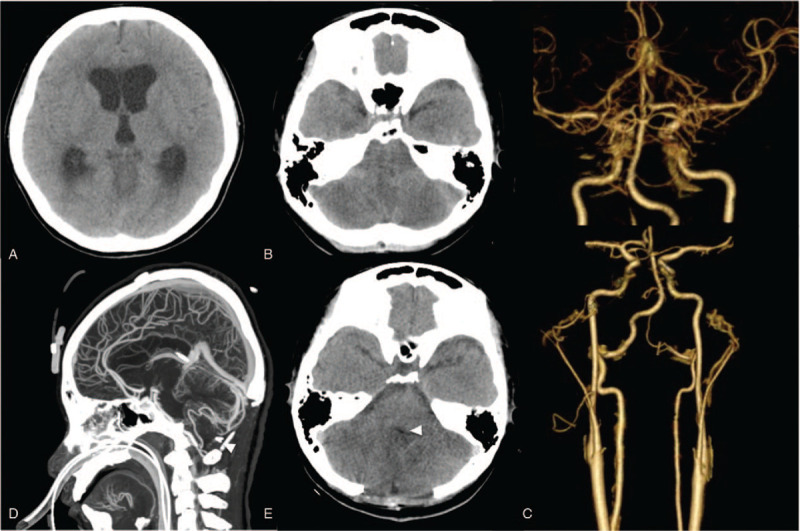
Pre- and postoperative brain CT/CT angiography. A preoperative brain CT showed severe obstructive hydrocephalus with ventriculomegaly (A) and low signal density of bilateral cerebellum with tight posterior fossa (B), indicating acute cerebellar infarction with brain stem compression. After emergent suboccipital craniotomy, a postoperative brain CT angiography was performed to investigate vascular patency (C–E). The anterior circulation was intact with vascular compromised; however, the left intracranial vertebral artery (VA) hypoplasia was noted and bilateral superior cerebellar arteries (SCA), anterior inferior cerebellar arteries (AICA), and posterior inferior cerebellar arteries (PICA) were vanished, suggesting a bilateral SCA, AICA, and PICA territories infarction. After craniectomy, the posterior fossa was decompressed till foramen magnum (D, arrowhead) and the fourth ventricle was patent (E, arrowhead). CT = computed tomography.
